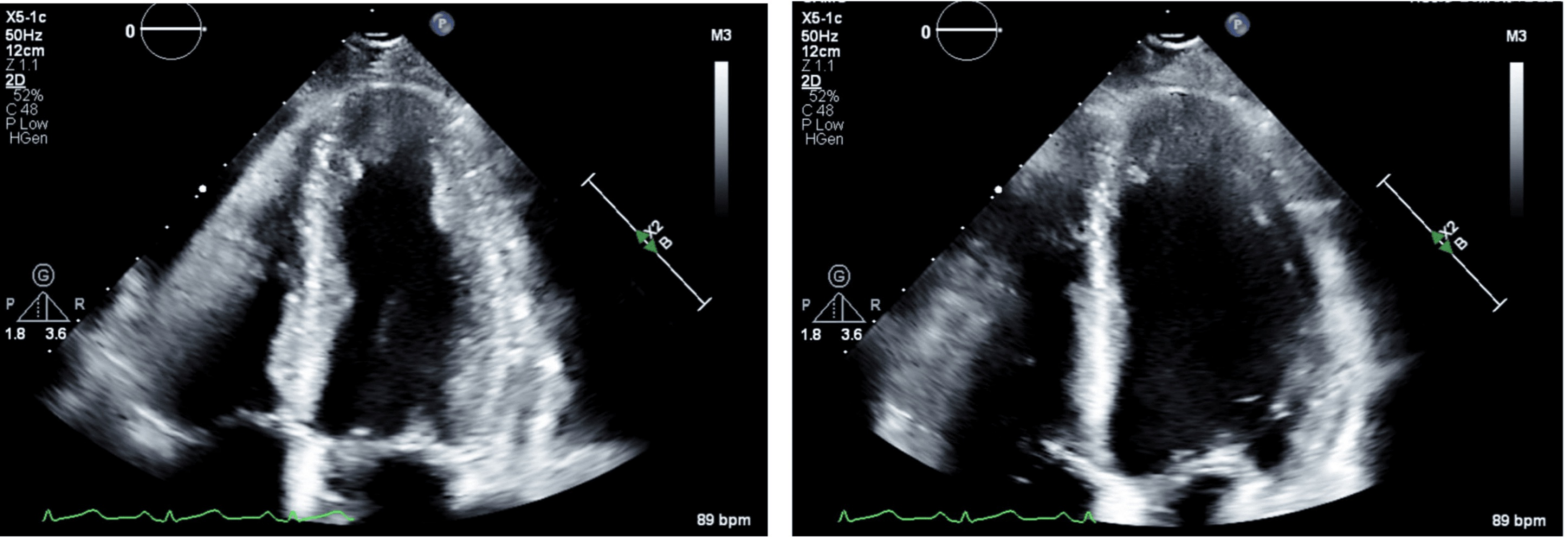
Apical four-chamber echocardiographic views demonstrating akinesis of the apex. The left panel shows the heart during systole, and the right panel during diastole, consistent with a regional wall motion abnormality.
